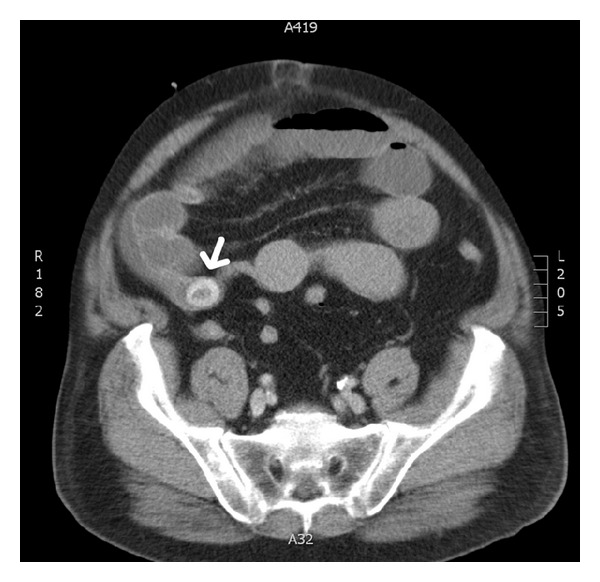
CT scan of the abdomen revealing 25 mm hyperdense lesion within the distal small bowel (white arrow).
